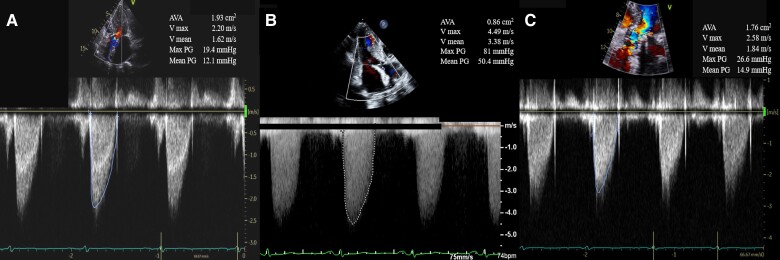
Changes in transthoracic echocardiography. (*A*) Predischarge transthoracic echocardiography findings (4 days following transcatheter aortic valve replacement). (*B*) Transthoracic echocardiography findings at exacerbation (54 days following transcatheter aortic valve replacement). (*C*) Transthoracic echocardiography findings after improvement (70 days following transcatheter aortic valve replacement).
